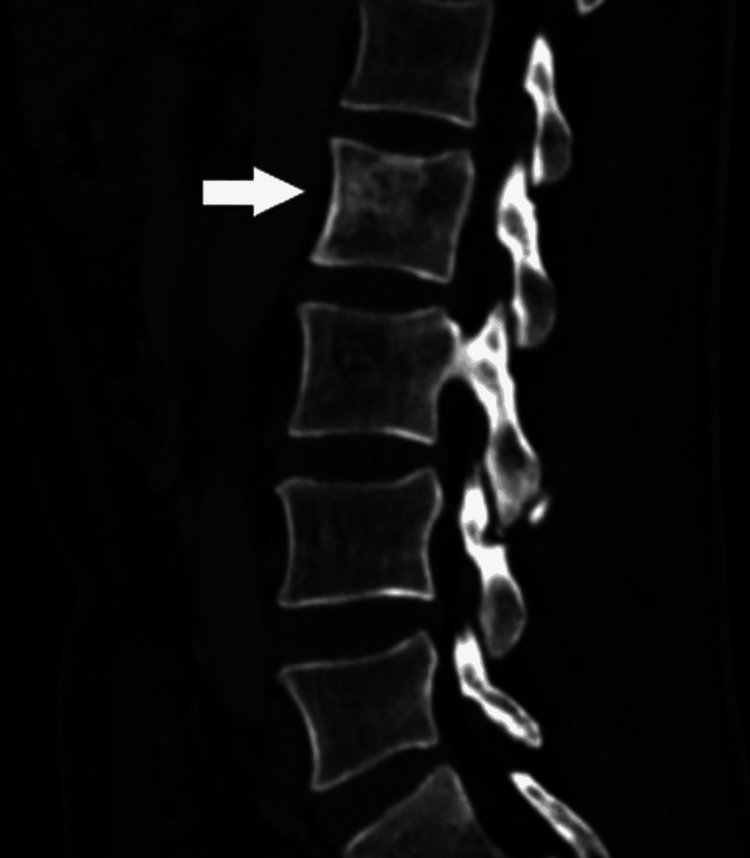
Sagittal abdominal CT scan showing lytic lesions of the vertebral body of the second lumbar vertebra.
